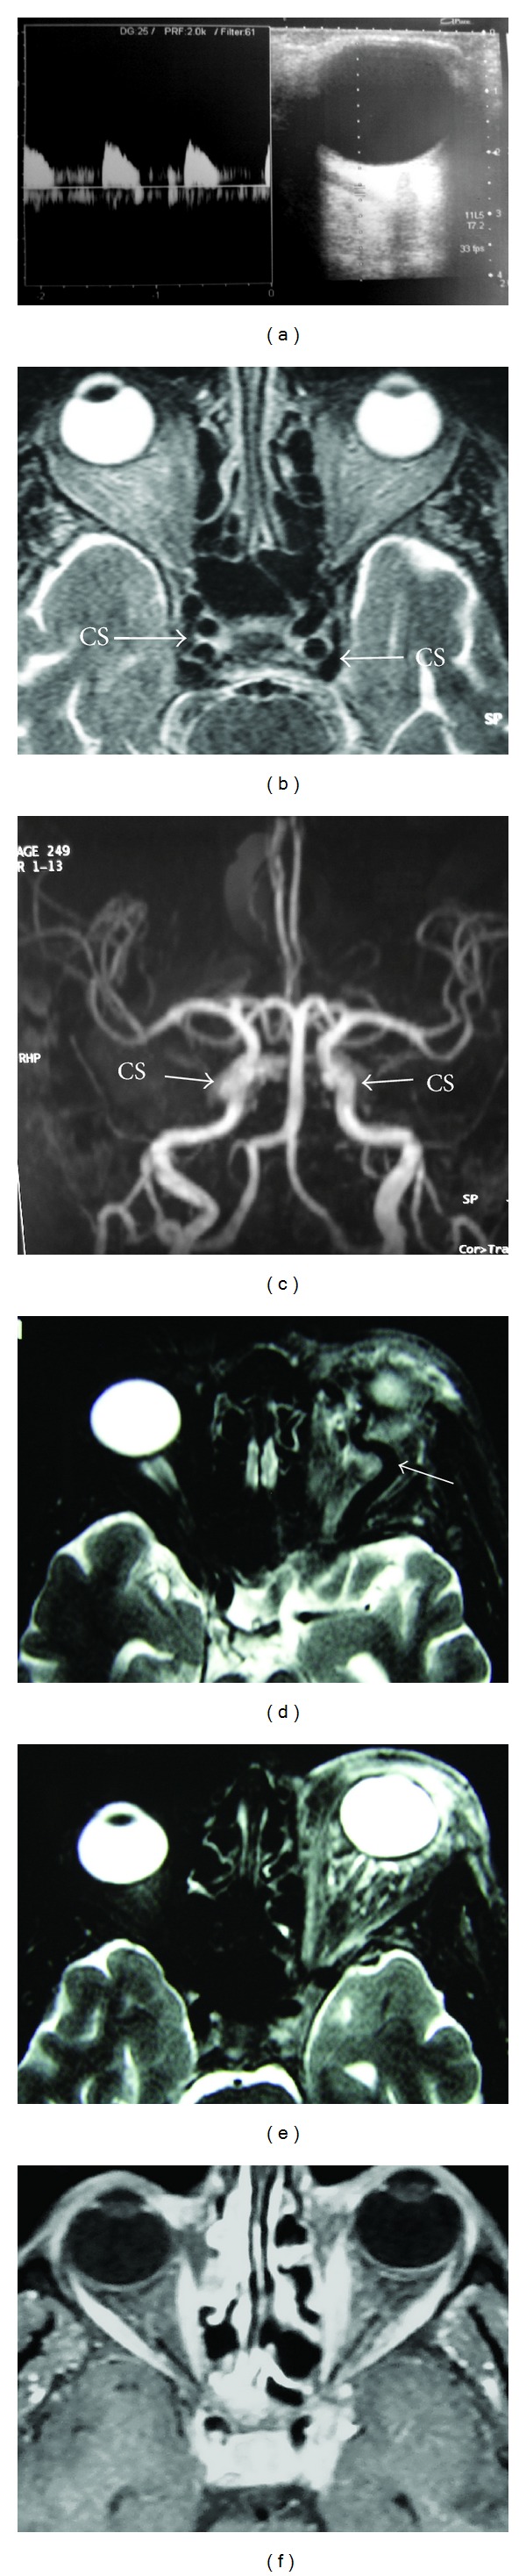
Imaging findings of CCF in different patients. (a) Arterialized flow in SOV on Doppler USG. ((b) and (c)) Arterialization of cavernous sinuses (CS) with flow void appearance on T2 weighted MRI (b) and flow related signal on maximal intensity projection MRA (c) in the same patient. ((d) and (e)) Left orbital congestion and exophthalmus with dilated SOV (arrow on d) and no evidence of flow void appearance of the left CS on T2 weighted MRI (e) but asymmetric intense enhancement of left CS (not shown here) in the same patient. (f) Asymmetric intense enhancement of left CS with no evident exophthalmus of left orbit nor arterialization of left CS on T2 weighted MRI or MRA but only dilated SOV (not shown here).
